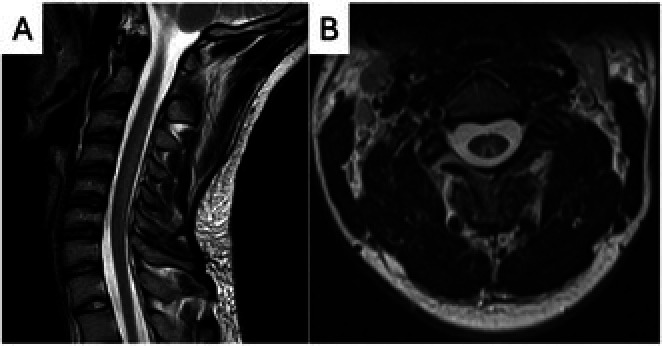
FIGURE 1 Cervical MRI in SCD. (A) Sagittal T2‐weighted of Patient 1: longitudinal hyperintense signal in posterior columns. (B) Axial T2‐weighted of Patient 4: bilateral and symmetric posterior column hyperintensity forming the “inverted V sign”.
